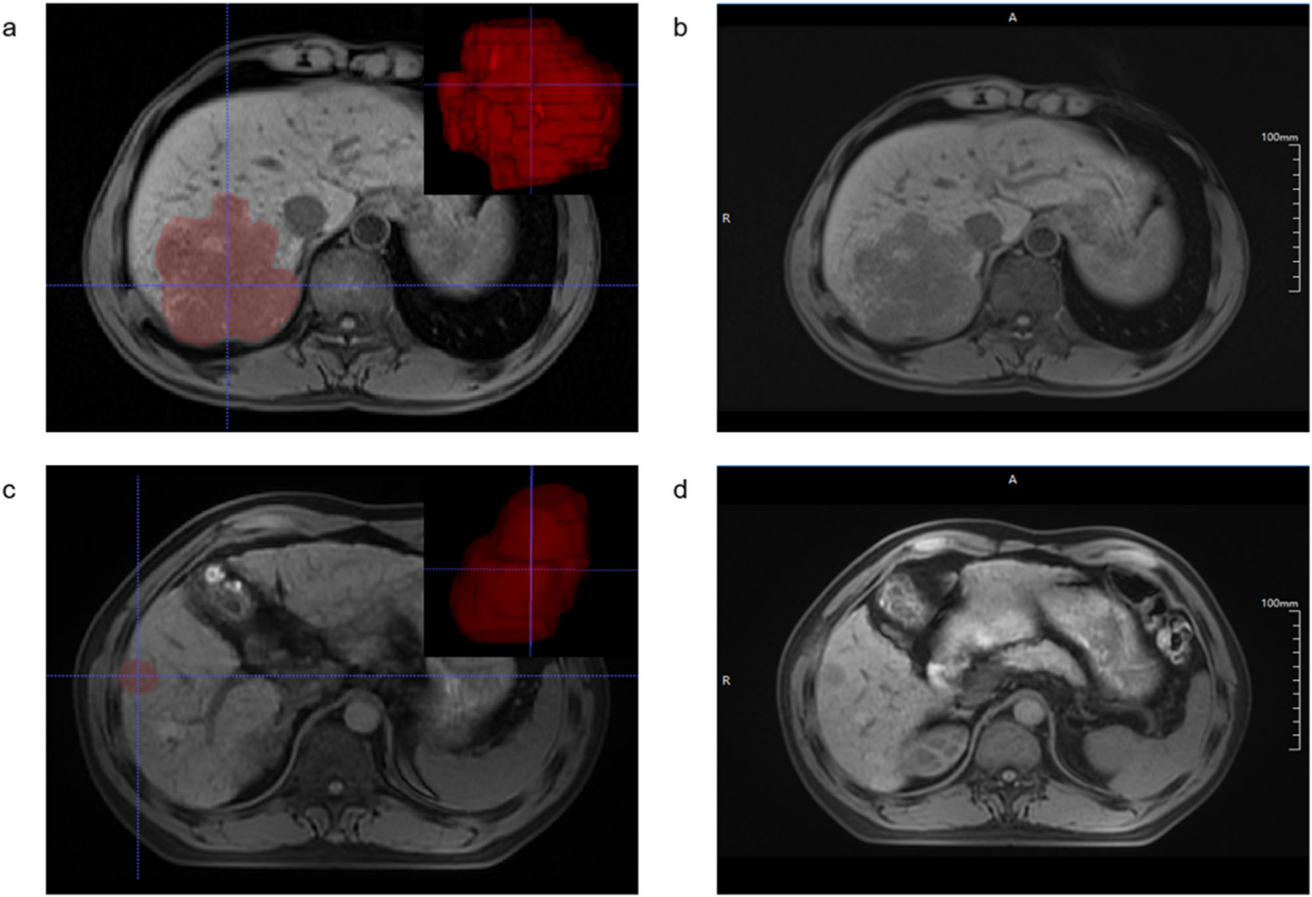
Two examples of applications for MVI status prediction in cHCC-CCA. a, b Images of a 46-year-old male with a 10.0 cm MVI-positive cHCC-CCA. Based on the radiomics model calculation, the radiomics score for this case is 0.928, and T1-weighted imaging shows homogeneous hypointensity of the lesion, with surface retraction (b). The predictive MVI status was positive. c, d Images of a 51-year-old male with a 2.5 cm MVI-negative cHCC-CCA. Based on the radiomics model calculation, the radiomics score for this case is 0.018, and T1-weighted imaging shows homogeneous hypointensity of the lesion, without surface retraction (d). The predictive MVI status was negative
